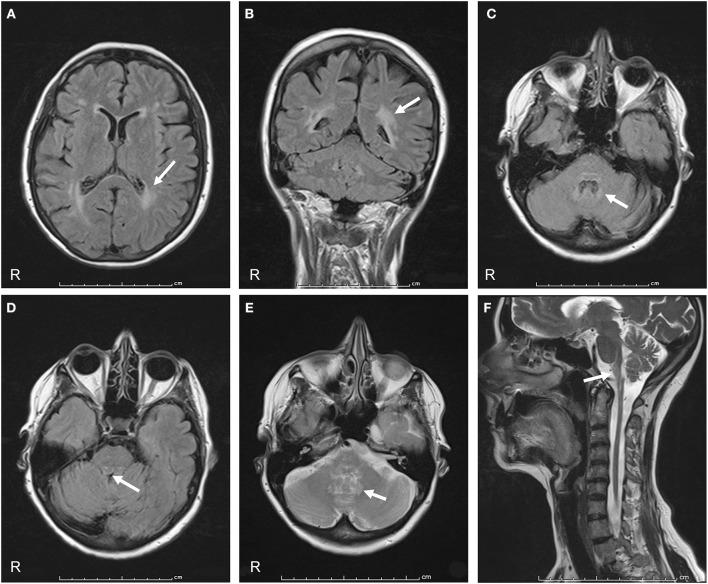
Axial and coronal FLAIR MRI show signal hyperintensity prevalent in the cerebral posterior periventricular regions (arrows in A,B) and in the hilum of the dentate nuclei (arrow in C). Midbrain and pons show peripheral rim of hyperintensity (arrow in D). Axial T2-weighted sections show increased signal intensity in the hilum of the dentate nuclei (arrow in E). A sagittal T2-weighted image shows atrophy and signal change in the medulla and spinal cord, “tadpole sign” (arrow in F).
